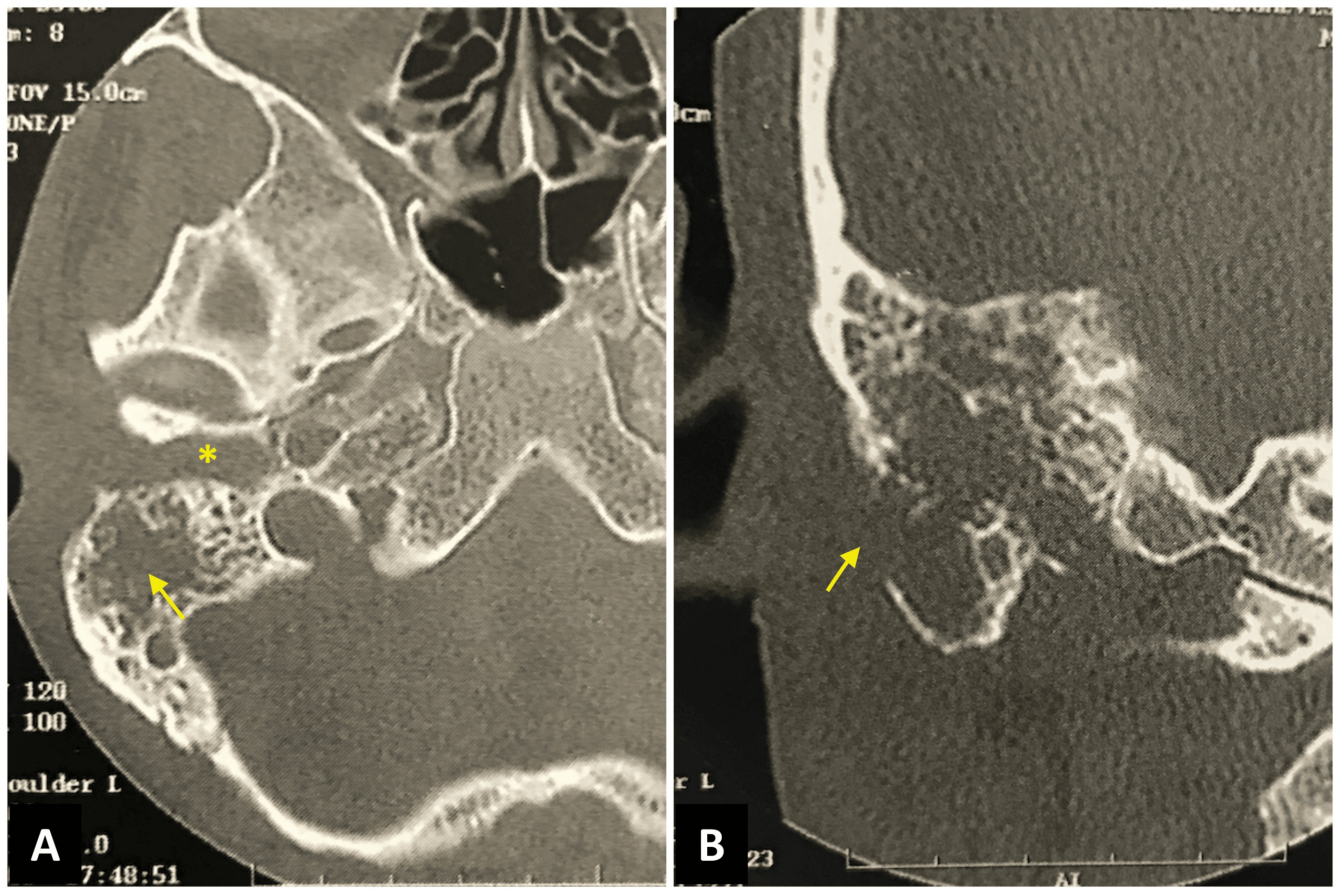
HRCT of the temporal bone detects coalescent mastoiditis on the right. (A) Axial section with erosion and bone sequestration of the mastoid trabeculae (arrow) and soft tissue density occupying the mastoid cavity, middle ear, and external auditory canal (asterisk); (B) coronal section showing discontinuity of the mastoid cortical layer (arrow). HRCT: high-resolution computed tomography
